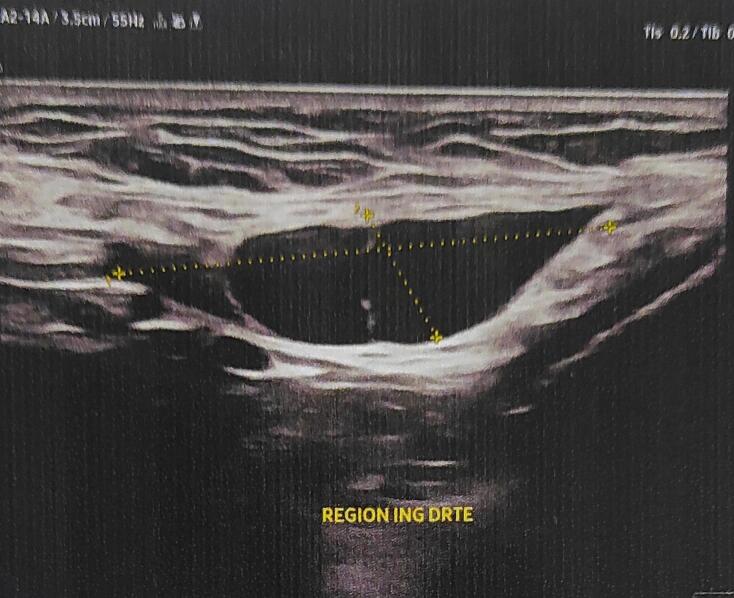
Ultrasound (US) that revealed a cystic structure of 4.1 × 2.3 cm in size with internal ipoecogenic mass.
